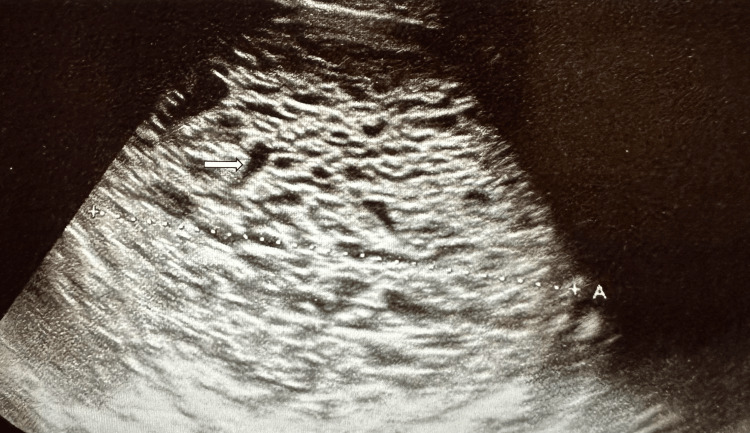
Transvaginal Ultrasound Showing a “Snowstorm” Appearance Ultrasound image of the uterus demonstrating the classic “snowstorm” pattern (white arrow), characteristic of a complete hydatidiform mole (CHM). The image shows diffusely enlarged, echogenic villous tissue without a viable fetus, consistent with the diagnosis of CHM.
